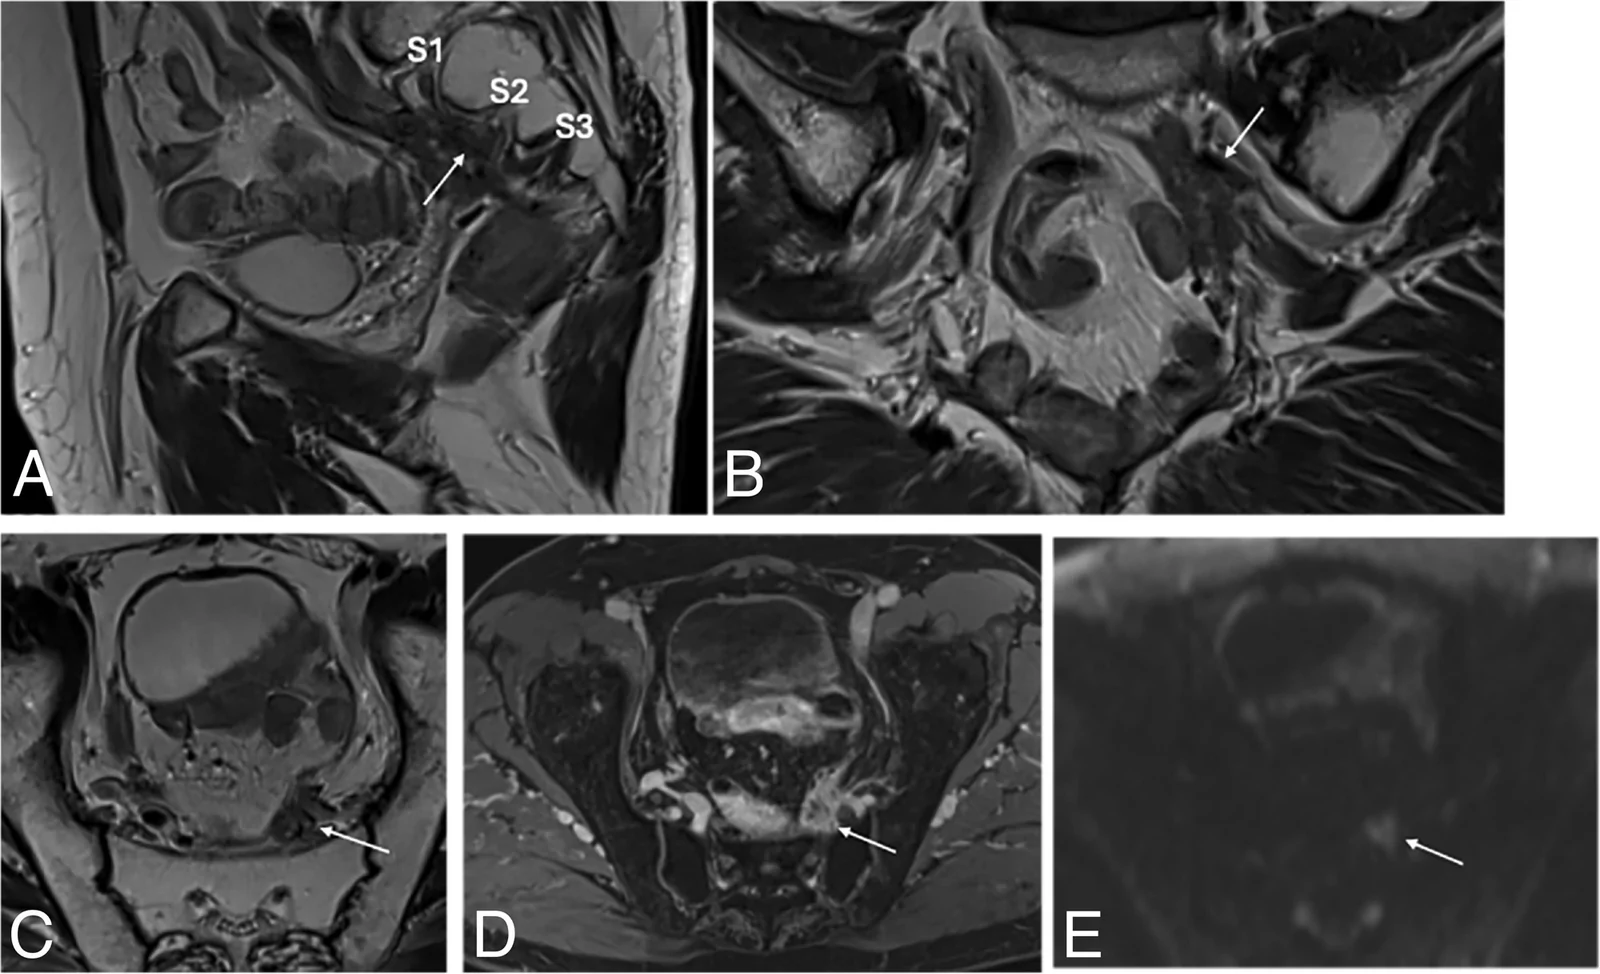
Sagittal (A), coronal (B) and axial (C) T2-weighted MR images of a female patient showing a recurrent rectal cancer in the posterior compartment following abdominoperineal resection. Note that the recurrence is difficult to depict on T2-weighted images and is better visualized on the post-contrast T1-weighted image (D) and DWI (E). The left S1-S2 nerve roots are involved. Key point: recurrence near upper sacral levels (S1–S2) is typically not resectable.
